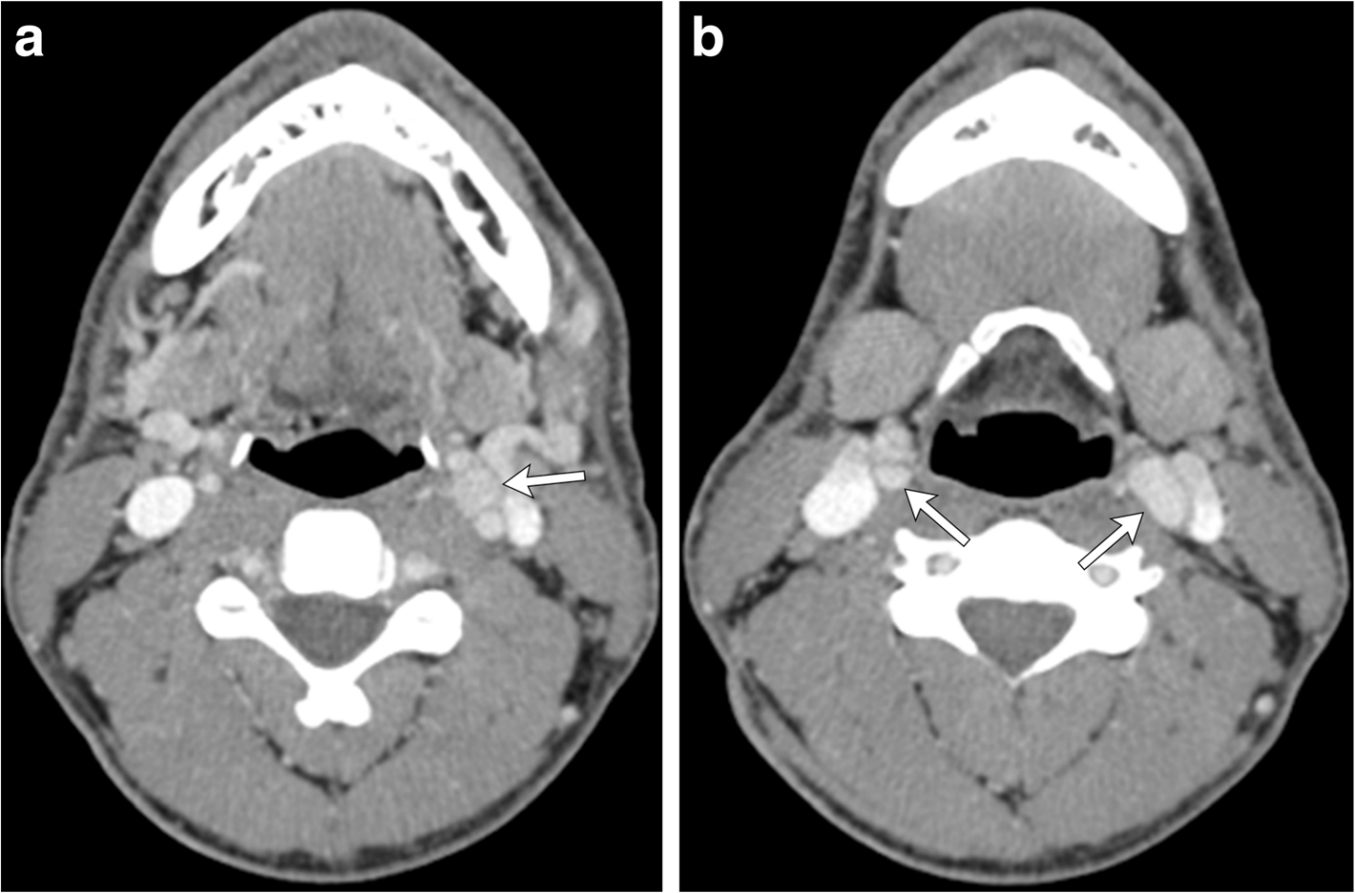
Axial contrast-enhanced CT images of the neck through superior (a) and inferior (b) portions of the carotid bifurcations demonstrate well-defined, avidly enhancing soft tissue masses (white arrows) within the bilateral carotid spaces, which splays the external and internal carotid arteries. Bilateral carotid body paragangliomas are much more common in patient’s living at high altitude
